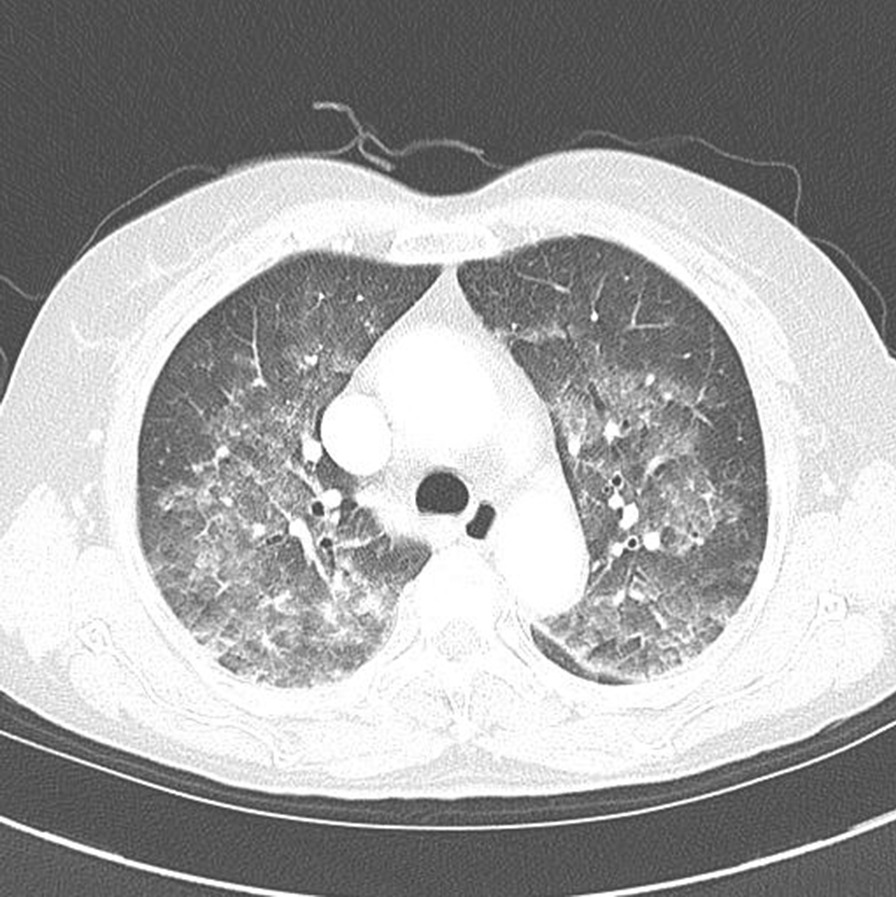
Computed tomography of the central portions of both lungs in the patient, showing diffuse ground glass opacity and interlobular septal thickening.
